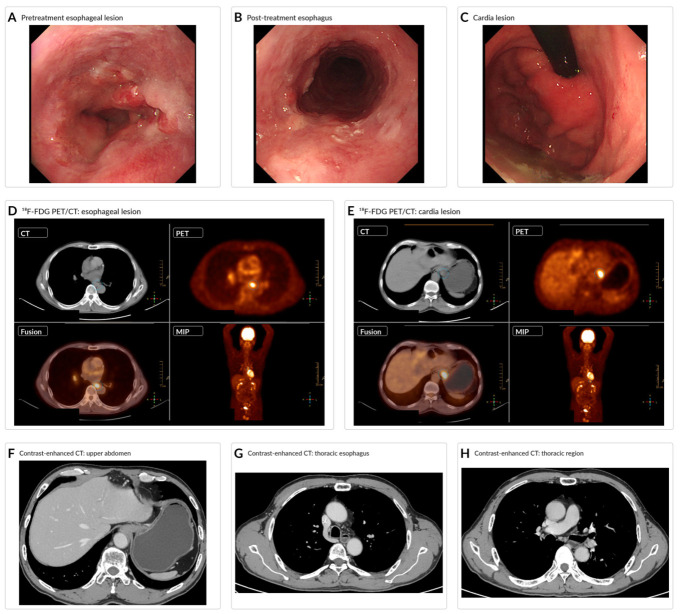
Endoscopic and radiological findings before and after treatment. (A) Pretreatment endoscopic appearance of the thoracic esophageal lesion. (B) Restaging endoscopy after one cycle of neoadjuvant chemoimmunotherapy, showing improvement of the esophageal lumen with residual mucosal abnormality. (C) Endoscopic appearance of the gastric cardia lesion. (D) Representative 18F-FDG PET/CT images of the esophageal lesion, including axial CT, axial PET, fused PET/CT, and maximum-intensity-projection views. (E) Representative 18F-FDG PET/CT images of the gastric cardia lesion in the corresponding four views. (F) Contrast-enhanced CT of the upper abdomen showing the cardia region. (G,H) Contrast-enhanced CT images of the thoracic esophagus and chest obtained for staging. No definite distant metastasis was identified.
